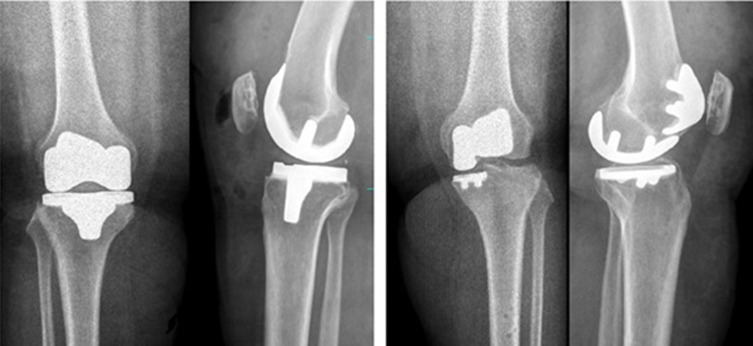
Post-operative knee X-rays of patients who underwent TKA (left-hand side) and BCKA (right-hand side).
